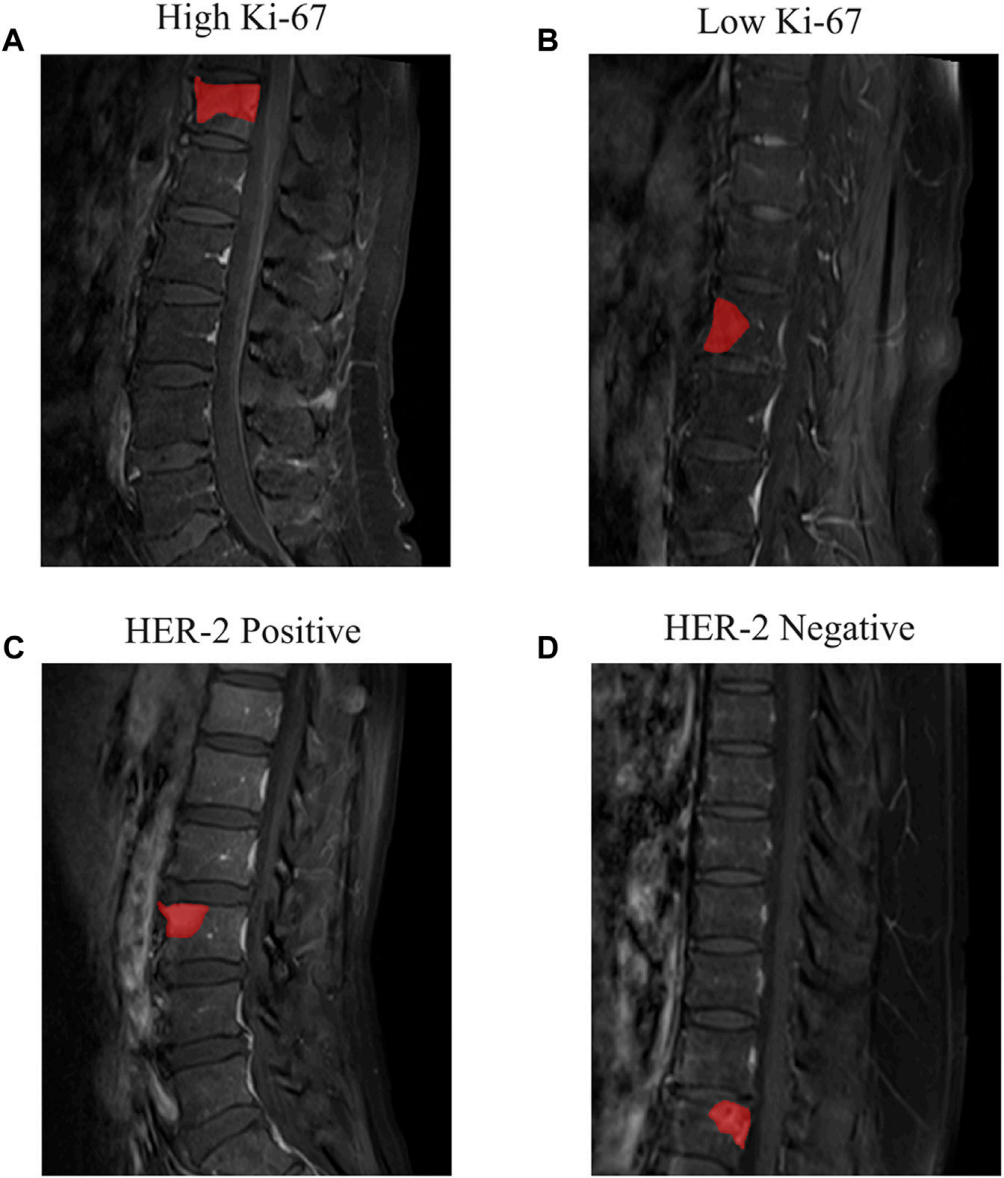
Examples of the T1CE MRI images of spine metastasis and segmented ROIs. (A) Patient with high Ki-67 expression level and (B) low Ki-67 expression level. (C) Patient with HER-2 positive and (D) HER-2 negative.
